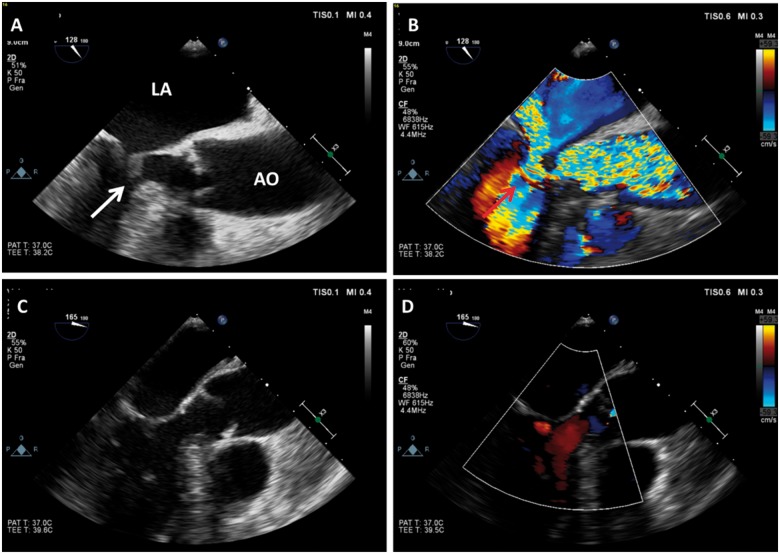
Transoesophageal echocardiographic systolic long-axis images. (*A* and *B*) Acquired before, and (*C* and *D*) Immediately after initiation of veno-arterial extracorporeal membrane oxygenation. (*A*) White arrow shows severe systolic anterior motion and (*B*) left ventricular outflow tract obstruction causing severe mitral regurgitation (red arrow). After initiation of veno-arterial extracorporeal membrane oxygenation systolic anterior motion, left ventricular outflow tract obstruction and mitral regurgitation disappeared.
